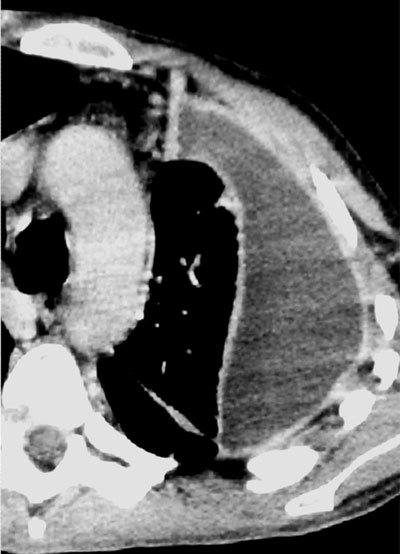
Split pleura sign – An axial contrast-enhanced CT view in mediastinal window at the level of the lung basis shows a pleural effusion with an enhancement and thickening of the visceral and parietal pleura.
